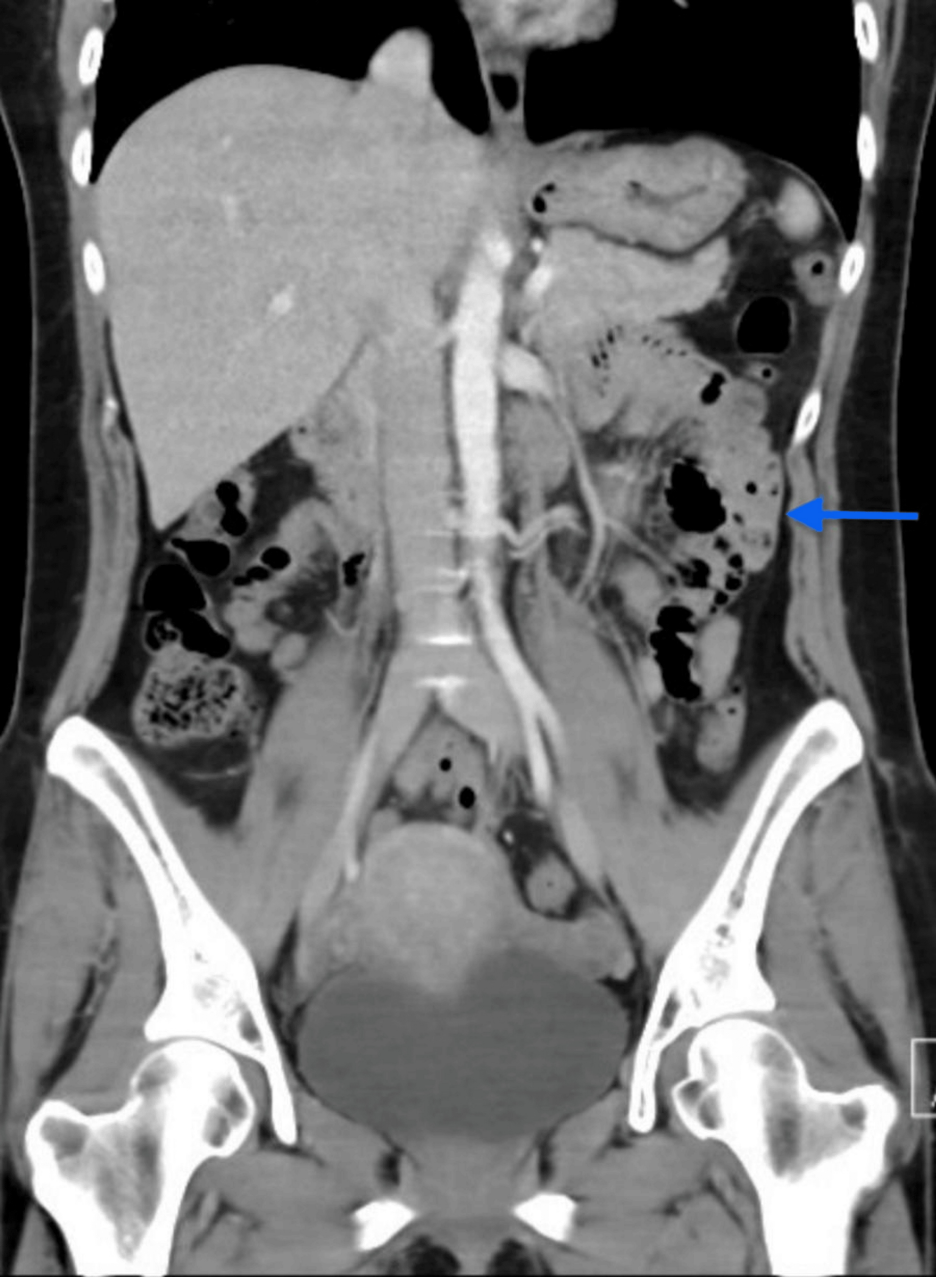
CT abdomen pelvis demonstrating pancolitis Bowel wall thickening and mucosal enhancement demonstrated by the blue arrow
